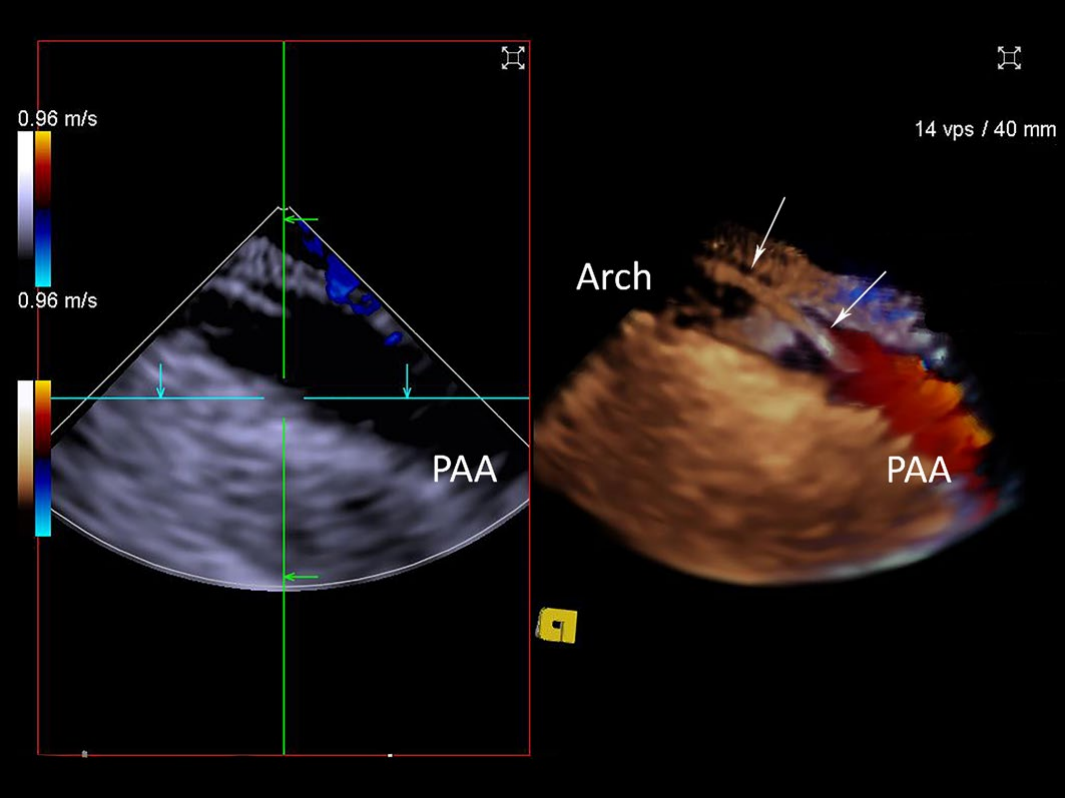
2D ICE (left panel) and 3D ICE Colour Doppler (right panel). Systolic aortic flow is demonstrated in the proximal aortic arch (PAA). The guidewire (white arrows) is clearly visualised within the aortic arch with 3D ICE imaging.
